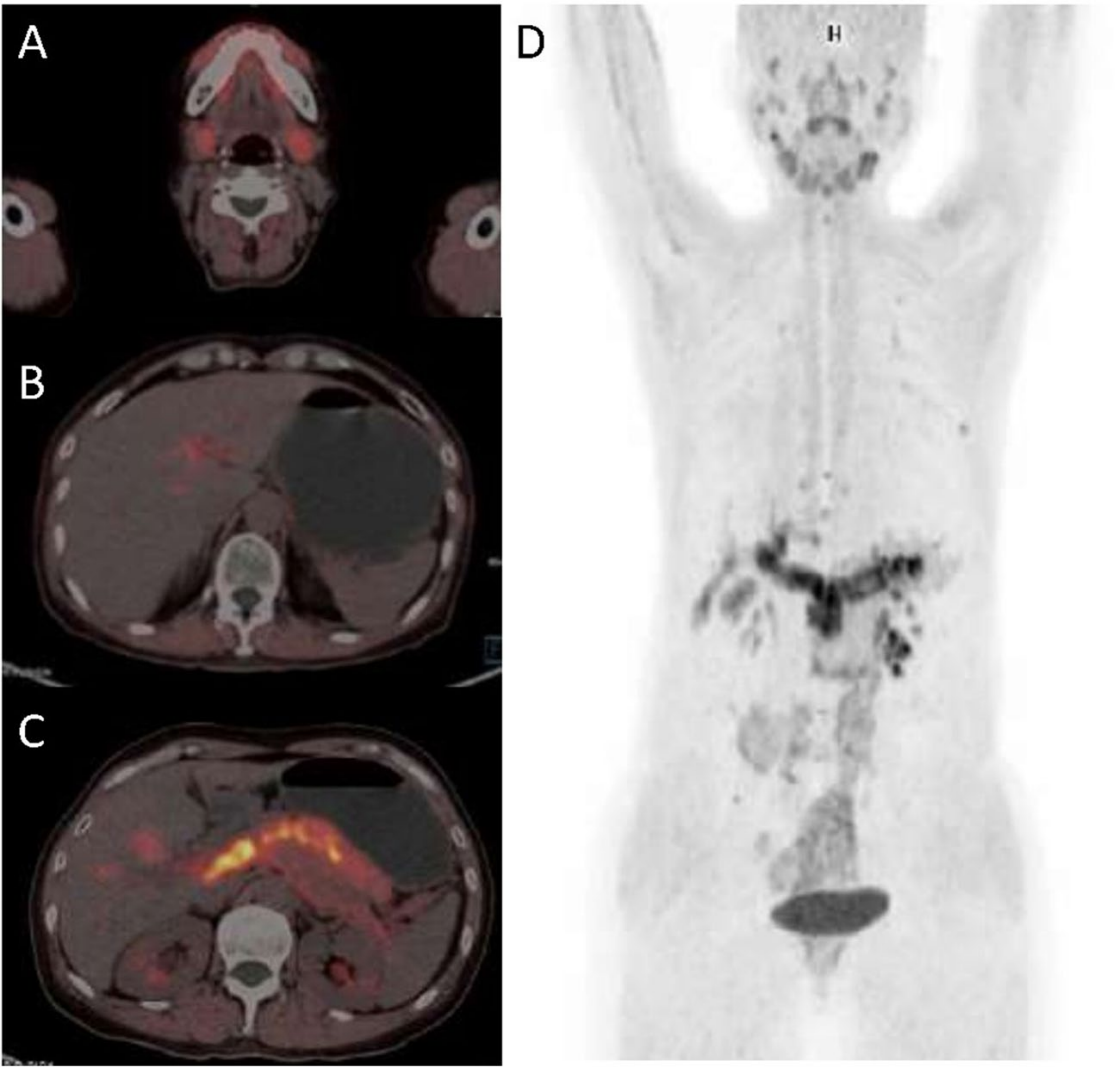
Hybrid PET/CT images of submandibular gland (A), bile duct (B), pancreas (C) and whole-body PET image (D), and from a 54-year-old female patient with IgG4-related disease. [^18^F]AlF-NOTA-FAPI-04 PET/CT shows increased [^18^F]AlF-NOTA-FAPI-04 uptake by pancreas (SUV_max_, 10.33), right lacrimal gland (SUV_max_, 6.04), left lacrimal gland (SUV_max_, 5.33), right submandibular gland (SUV_max_, 7.14), and left submandibular gland (SUV_max_, 8.58). SUV, standardized uptake value
